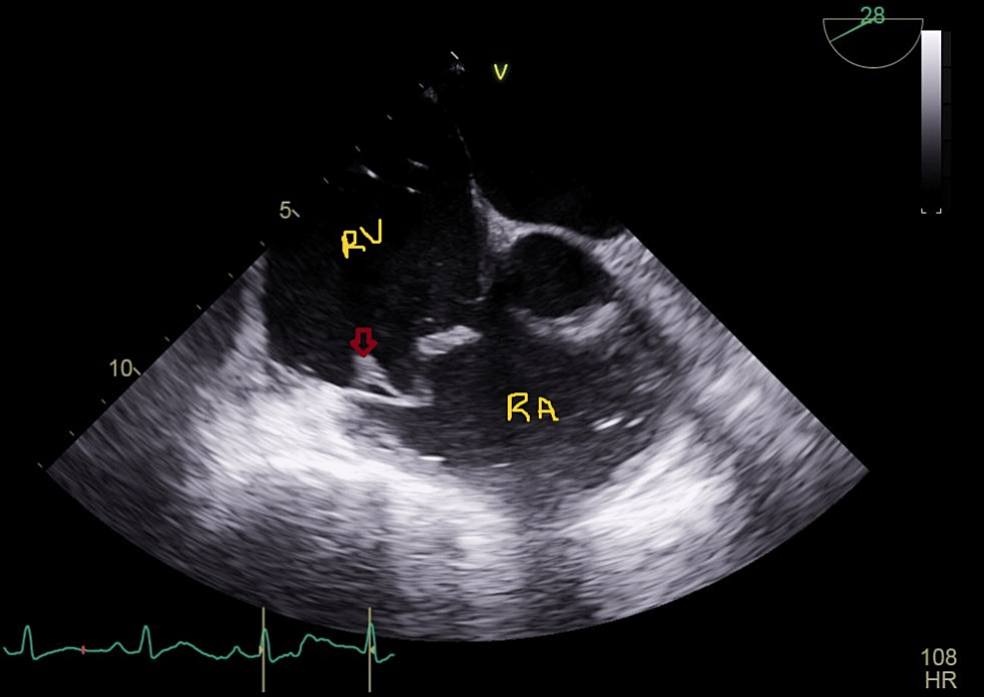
Transthoracic echocardiogram showing mobile vegetation on the tricuspid valve labeled with a red transparent arrow. The right atrium and right ventricle are labeled as RA and RV, respectively, in yellow initials. RA: Right atrium, RV: Right ventricle, HR: Heart rate
